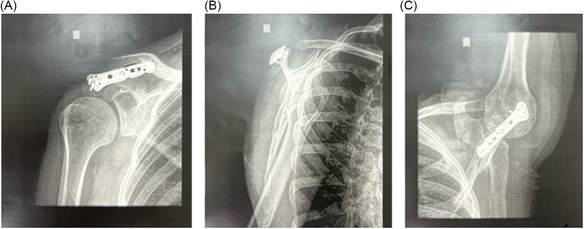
Postoperative radiographic images demonstrating acromial fixation using a distal radius locking plate. (A) Anteroposterior view showing the position of the plate along the posterior acromion. (B) Scapular Y view confirming proper posterior translation and alignment, without causing acromioclavicular joint issues or displacement. (C) Axillary lateral view demonstrating stable fixation and increased posterior acromial coverage over the humeral head.
